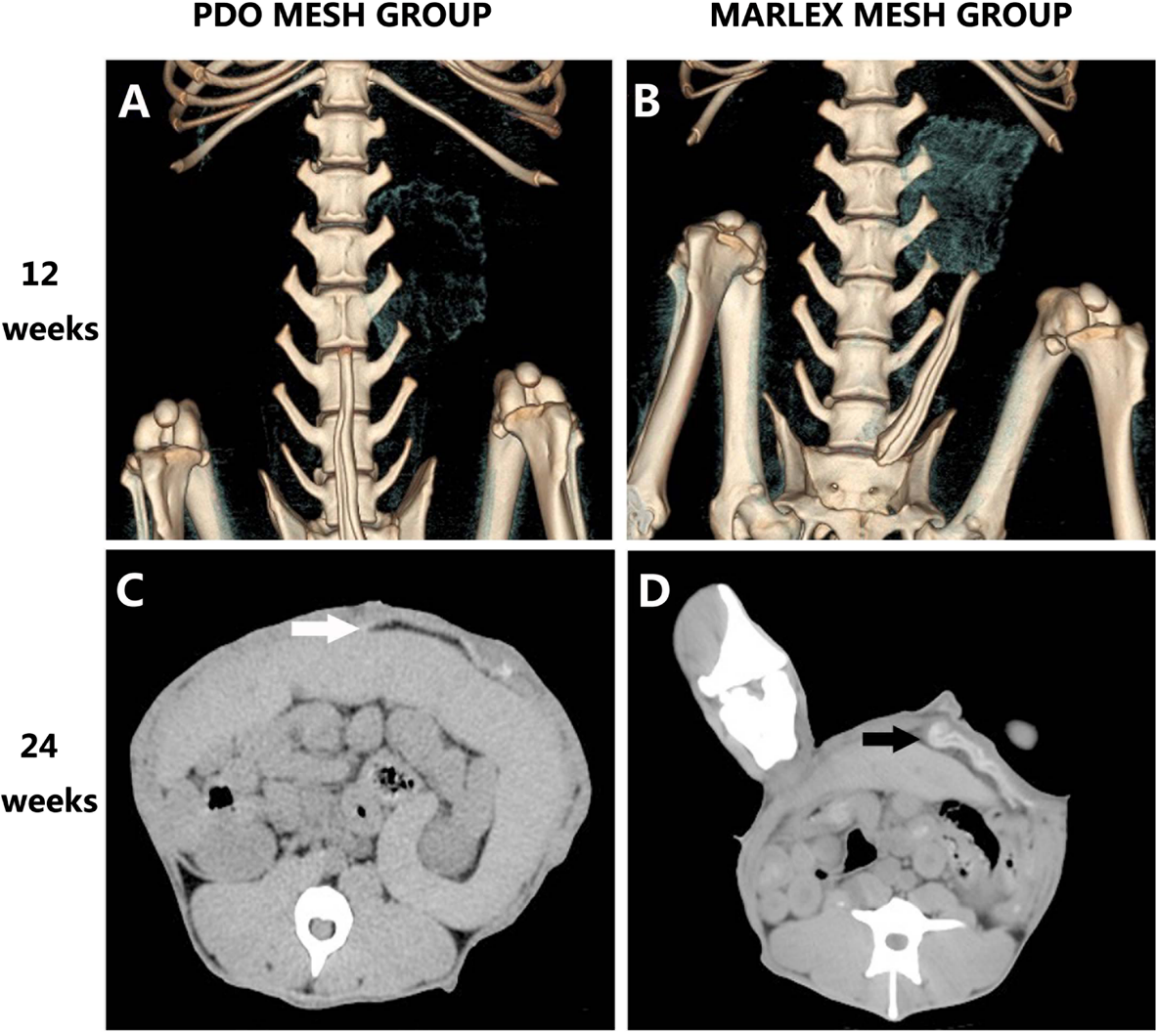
Three-dimensional reconstructions of abdominal computed tomography scans. At 12 weeks, The poly-p-dioxanone (PDO) mesh (A) and the Marlex mesh (B). At 24 weeks, On abdominal CT scans, the poly-p-dioxanone (PDO) mesh (black arrow in (C)) and the Marlex mesh (white arrow in (D)).
